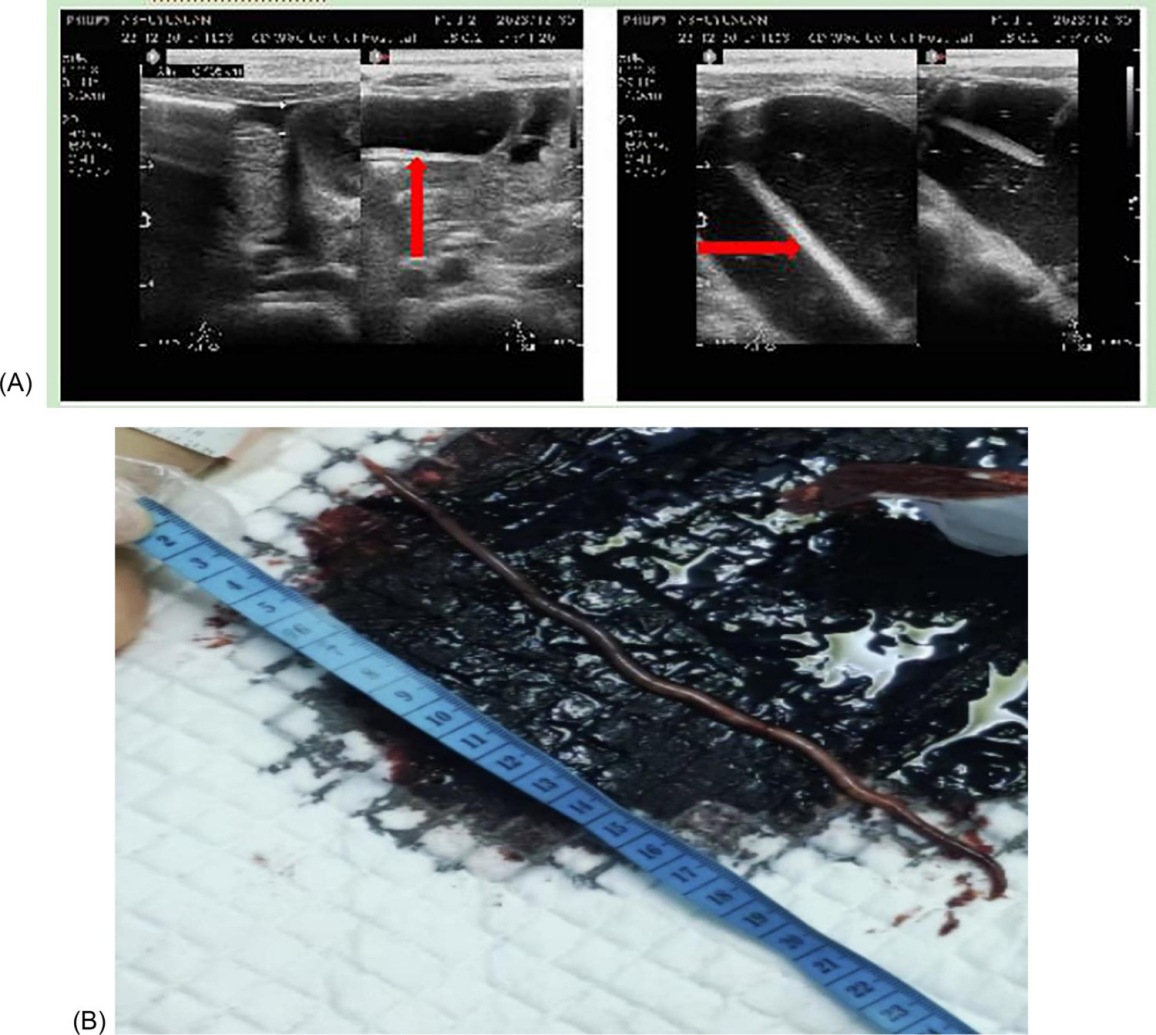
(A) Abdominal ultrasound showing *A. lumbricoides* (red arrow) as a linear hyperechoic structure. (B) A 21 cm adult worm was passed per rectum.
